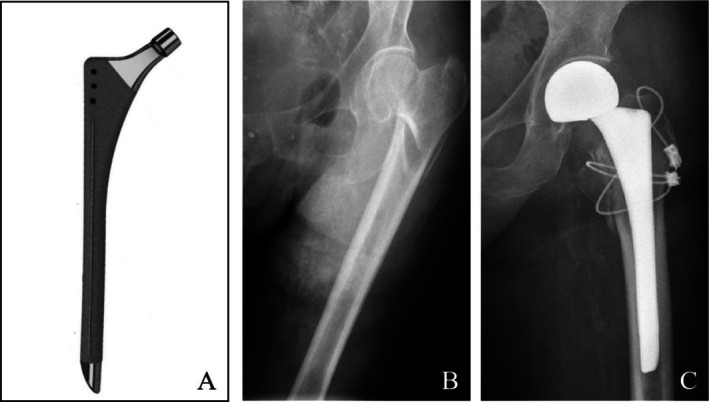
(A) The stem used in this study was a fully hydroxyapatite‐coated long stem. (B) Female, 93 years old, the anterior–posterior radiograph showed an intertrochanteric fracture. (C) The anterior–posterior radiograph after hip arthroplasty, the dissociative lesser trochanter and the greater trochanter were fixed by two cables.
